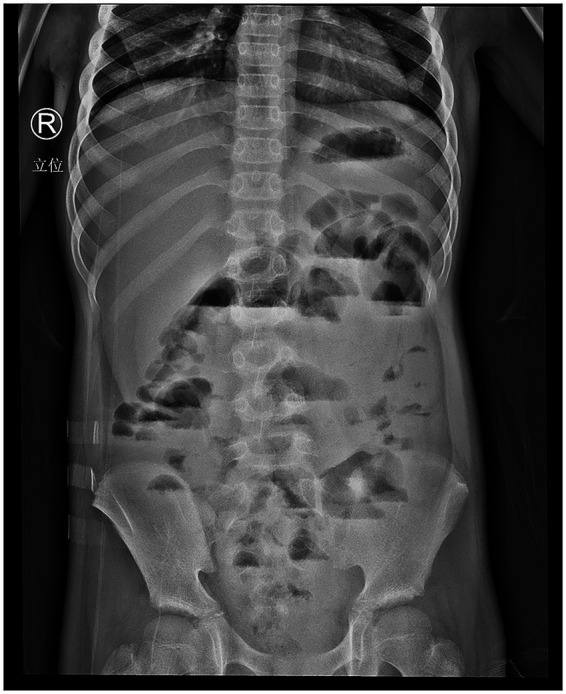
Abdominal X-ray examination revealed intestinal obstruction. The middle and upper abdominal parts of the intestine revealed gas accumulation, expansion, and multiple liquid–gas planes with different heights.
